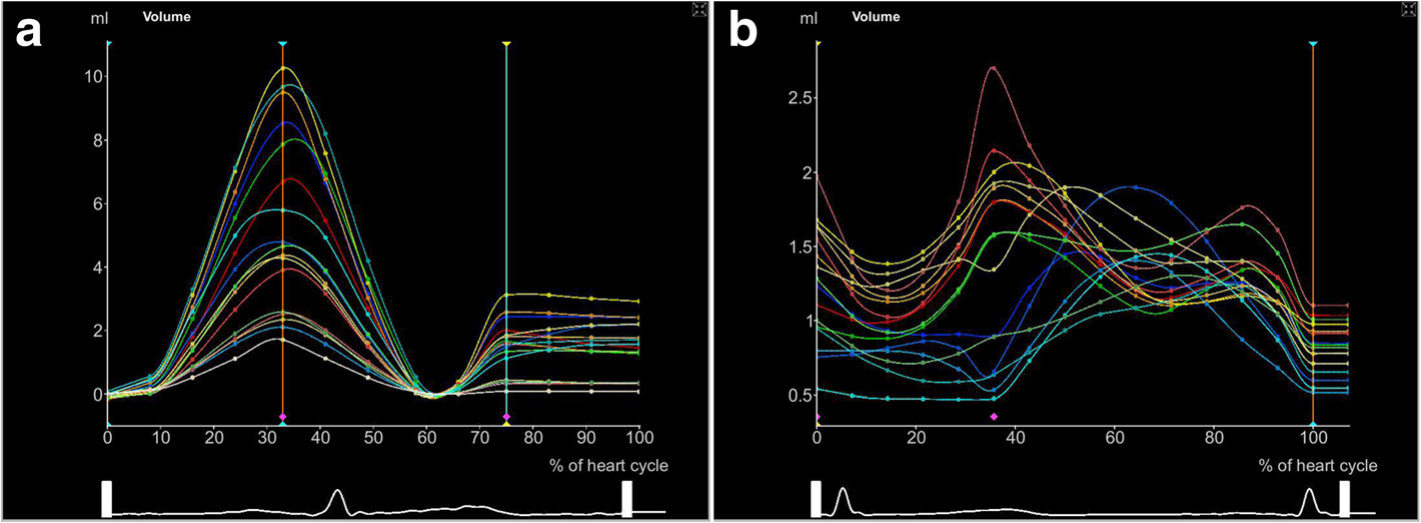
The regional left ventricular volume–time curve of healthy controls (a) and patient cases (b) by real-time three-dimensional echocardiography (RT-3DE)
